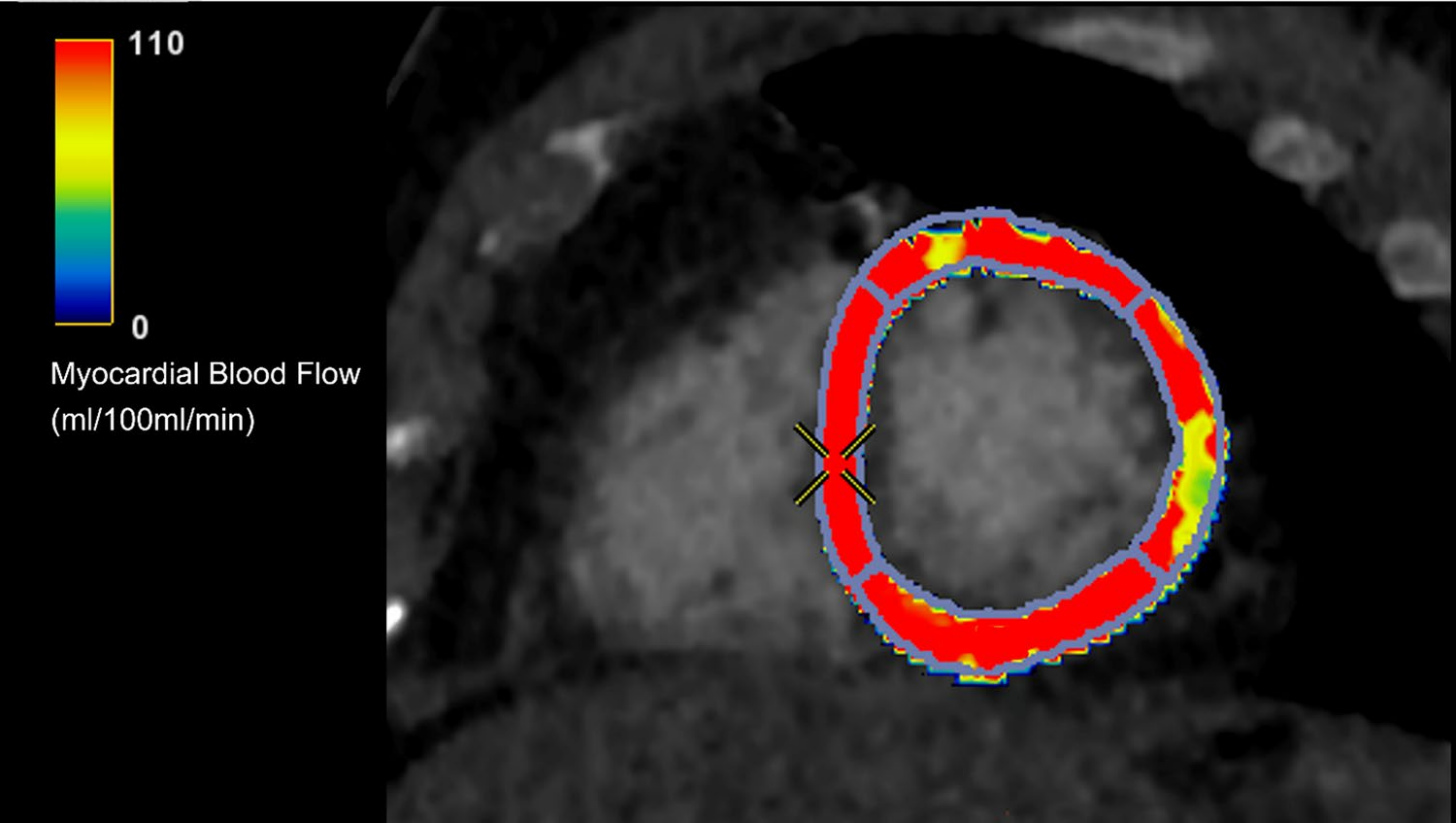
Short axis color-coded parametric map of myocardial blood flow obtained during stress myocardial perfusion CT after injection of Regadenoson showing a perfusion defect in the middle lateral segment of the left ventricle
